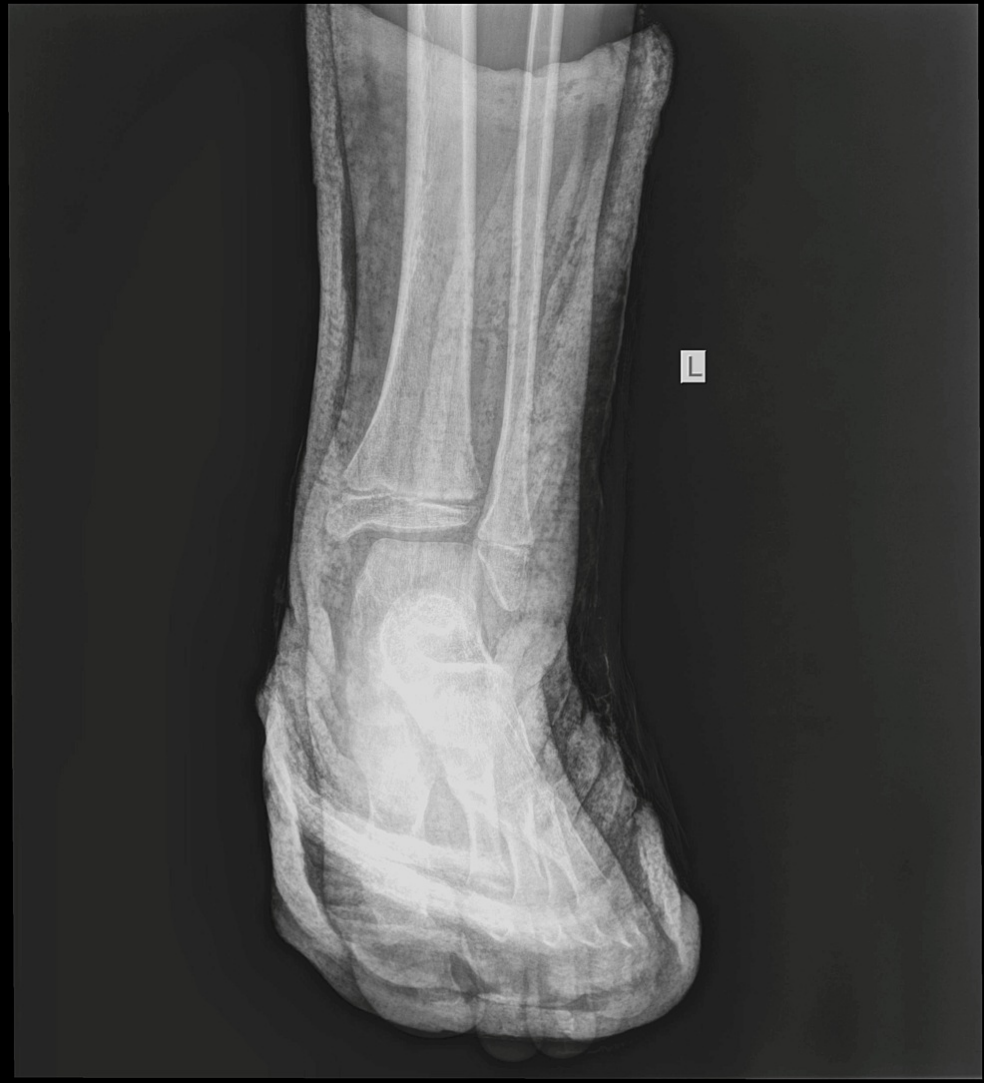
X-ray AP, after immobilization in a cast
